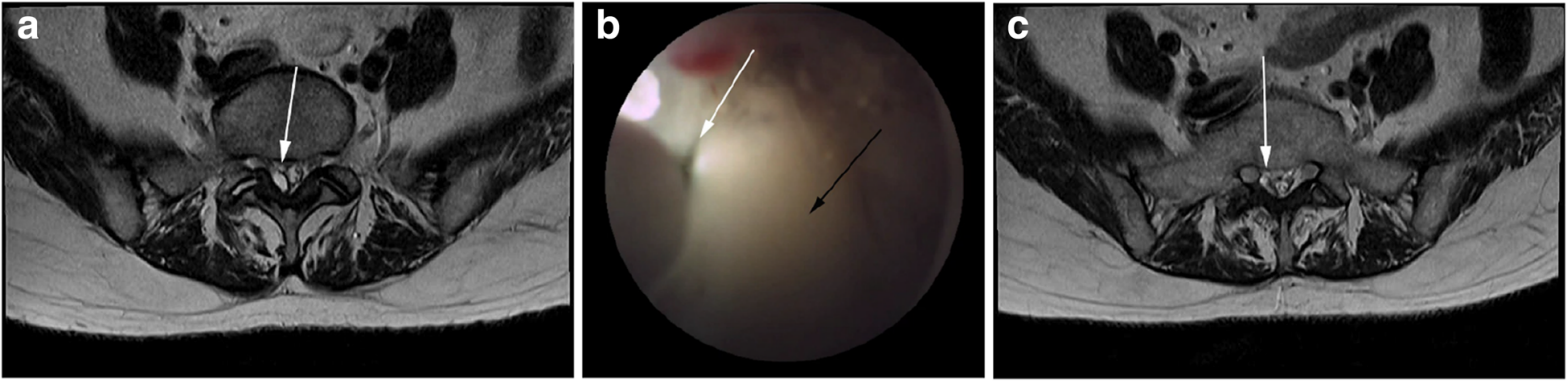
a Pre-operative magnetic resonance (MR) image shows a facet-originated cyst (arrow) compressing the thecal sac sacral (S) 1 nerve root. b Epiduroscopic image shows the laser electrode inserted into the cystic mass (white arrow); the mass decreased in size (black arrow) post-surgery. c Post-operative MR image shows the decompressed cyst (arrow) and the released thecal sac and S1 nerve root
